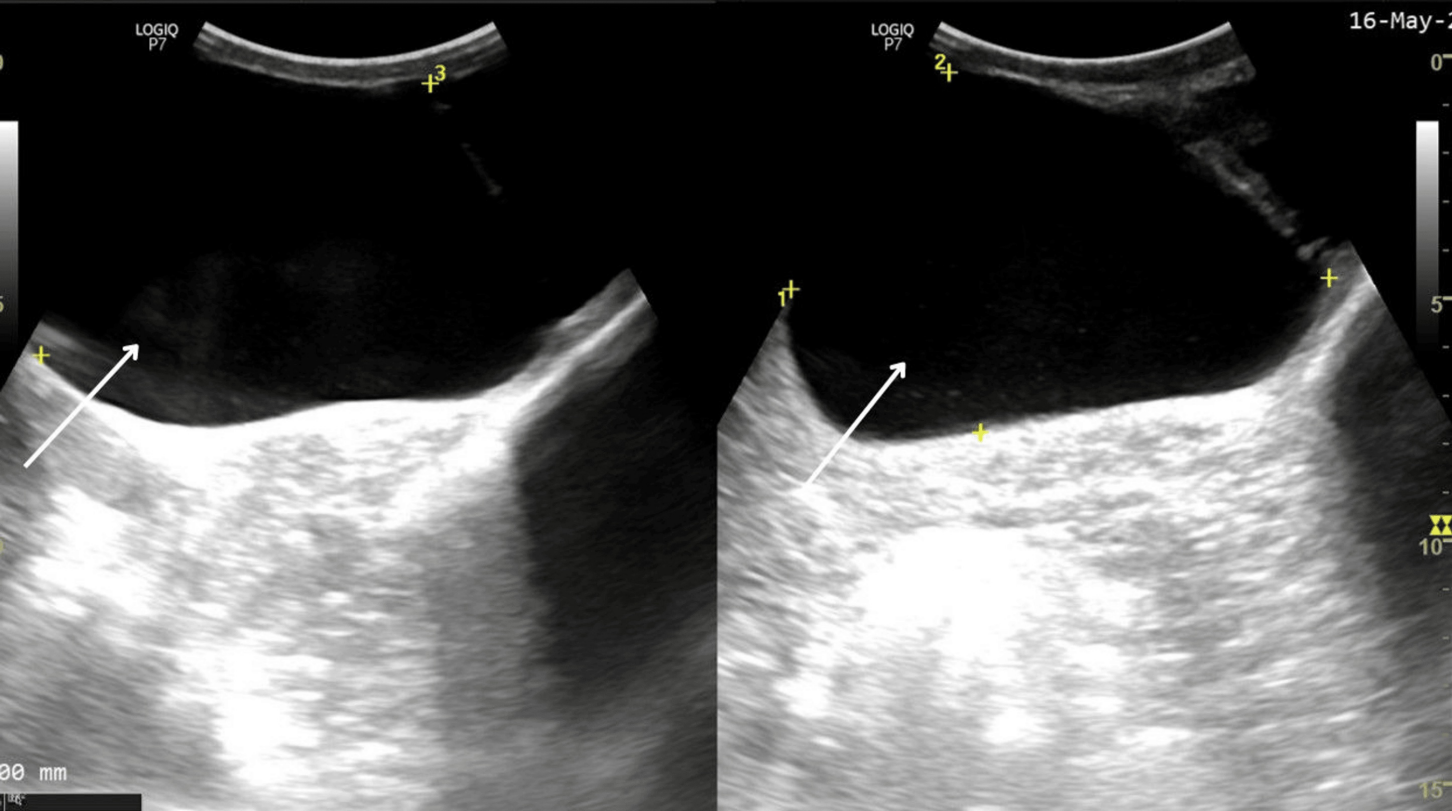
Ultrasound showing the cyst (marked with white arrow)
